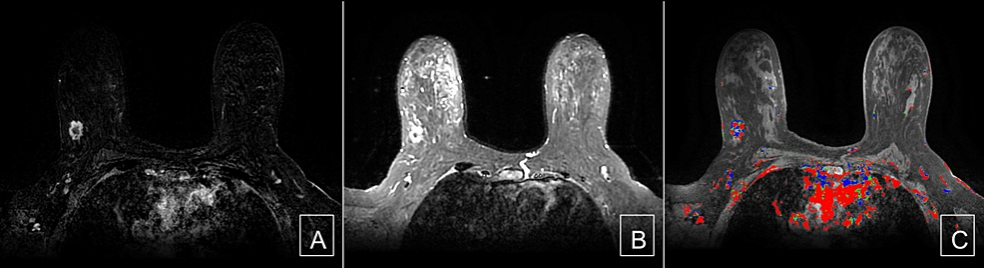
Cancer: subtracted image, T2 image, and kinetics image (A) A fat-saturated subtracted T1 weighted post-contrast image demonstrates irregular mass with irregular margins with heterogeneous internal enhancement. (B) A fat-saturated T2-weighted image demonstrates a high signal within the invasive ductal carcinoma. (C) A fat-saturated T1-weighted post-contrast with kinetic overlay demonstrates predominantly rapid washout kinetics.
